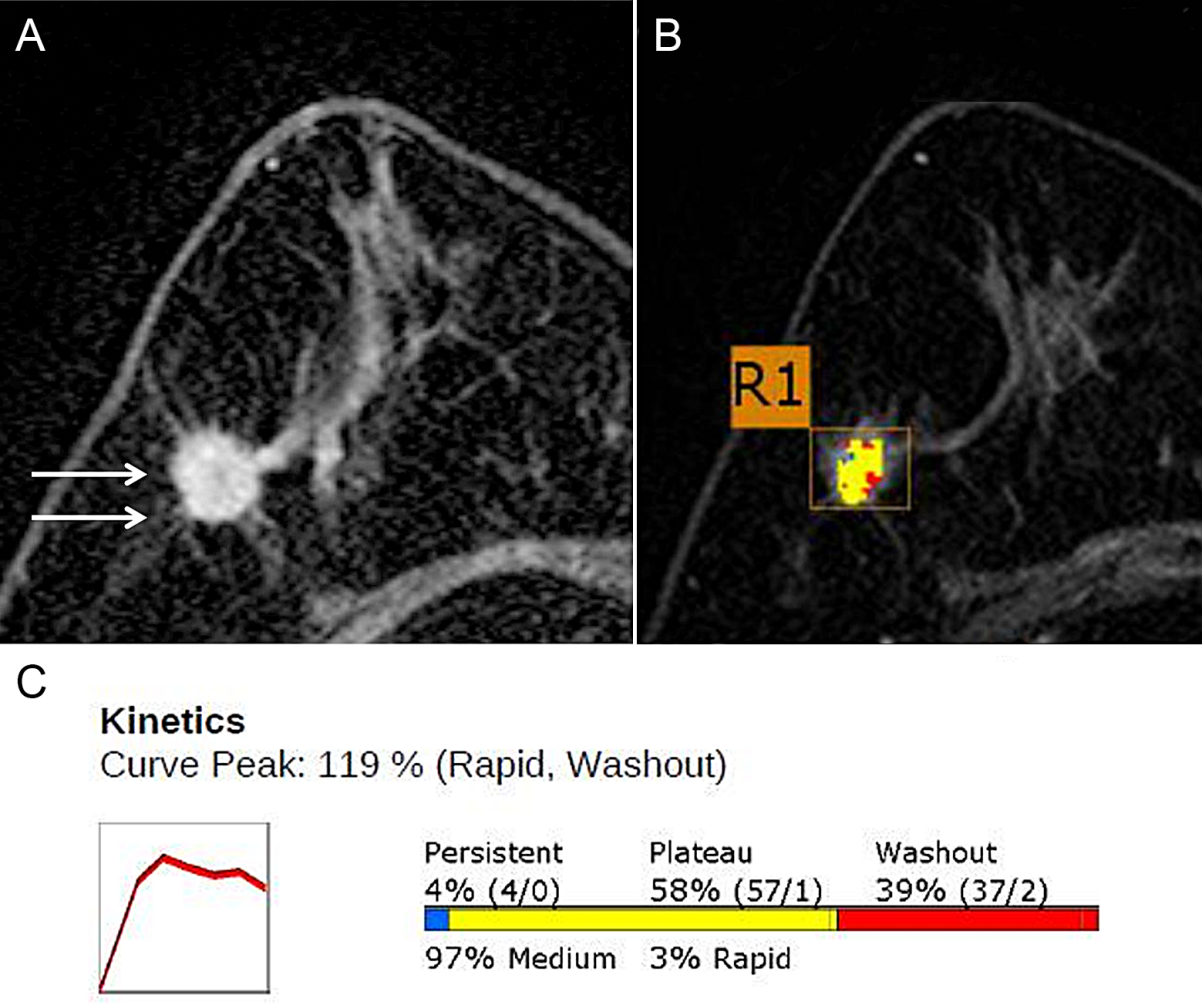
Magnetic resonance (MR) Images with Computer-aided detection (CAD) Color Overlay Map in a 71-year-old Woman with grade 2 invasive ductal carcinoma in the right breast. (A) Axial contrast-enhanced T1-weighted MR image shows a 15-mm irregular mass (arrows). (B) Axial maximum-intensity-projection MR image shows a CAD color overlay over the breast mass. R1 denotes the first lesion of the right breast. Areas in red, yellow, and blue indicate rapid washout-type delayed enhancement, plateau-type delayed enhancement, and persistent-type delayed enhancement patterns, respectively. (C) Graph of the kinetic curve shows rapid initial enhancement and rapid washout-type curve. The initial peak enhancement value was 119%. With respect to the delayed phase enhancement, 39% of the mass showed washout, 4% of the mass showed a persistent-type curve, and 58% showed a plateau-type curve. There was no recurrence during the 65-month follow-up period.
